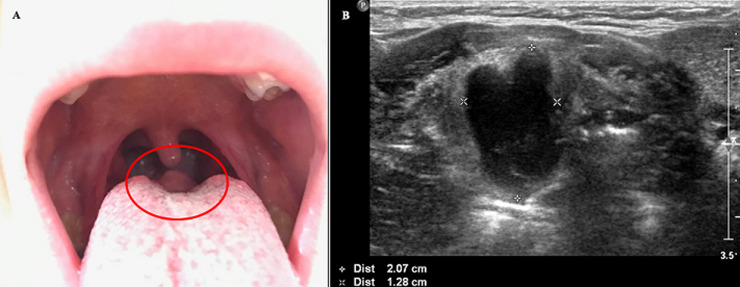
A) tumour in the posterior base of the tongue, compatible with lingual TDC; B) ultrasonographic appearance of the lesion, adjacent to the blind foramen of the tongue
